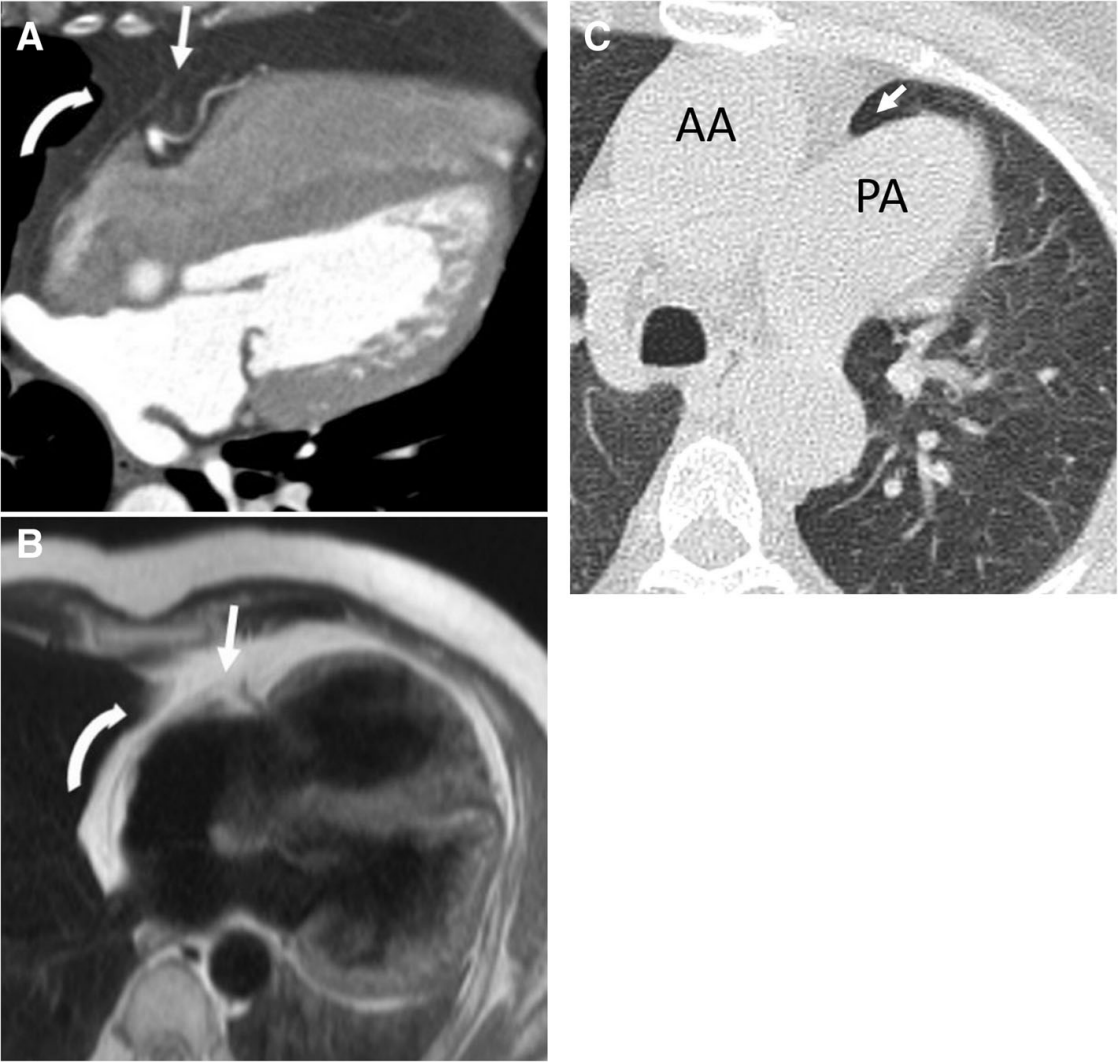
A 51-year-old man. Axial contrast-enhanced CT (a) and T2-weighted MR images (b) demonstrate levorotation (curved arrows) of the heart and lack of pericardial continuity (arrows). c Axial CT image of a different patient with congenital absence of the pericardium demonstrates a prominent main pulmonary artery (PA). Interposed lung tissue (arrow) between the main pulmonary artery and the ascending aorta (AA)
